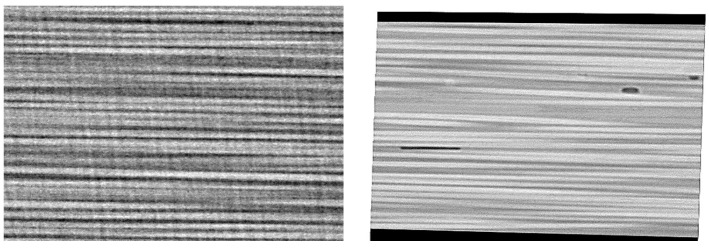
Samples of the two-dimensional (2D) images obtained through the fibre direction from the three-dimensional (3D) images resulting from the CT scan.
